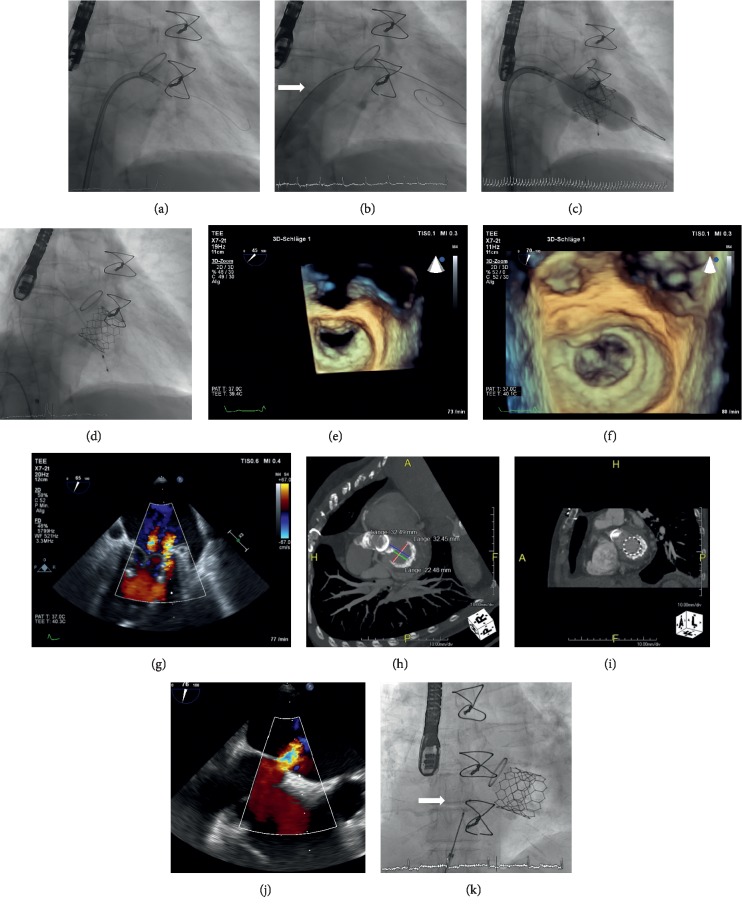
TMVI-MAC: (a) fluoroscopy shows a 0.035-inch J-guide inserted through the mitral valve in the left ventricle via a steerable guiding catheter (Agilis, 8,5F, Abbott, Illinois, USA). (b) Fluoroscopy shows balloon dilatation (Osypka VACS II 12 × 60 mm Balloon, Osypka AG, Germany, white arrow) of the interatrial septum over an Amplatz Super Stiff guidewire (Boston Scientific) to create an artificial atrial septum defect prior to insertion of the prosthesis into the left atrium. (c) *Fluoroscopy* shows the implantation of a 29 mm Edwards SAPIEN 3 (ES 3) prosthesis into the heavily calcified native mitral valve. (d) *Fluoroscopy* shows the final result after implantation of the ES 3 prosthesis into the calcified native mitral valve. (e) 3D-*TEE* shows the native mitral valve with a heavily calcified native mitral ring prior to TMVI (view from left atrium). (f) 3D-TEE shows the result after TMVI of the 29 mm ES 3 prosthesis into the advanced mitral annular calcification (MAC). (g) 2D-*TEE* with color duplex shows only minimal paravalvular and valvular regurgitation after implantation of the ES 3 Prosthesis into the MAC. (h) Cardiac CT-scan shows the exact dimensions of the calcified mitral valve prior to implantation. (i) Cardiac CT-scan shows the final result after TMVI into MAC. (j) 2D-TEE with color duplex shows a large iatrogenic atrial septal defect (ASD) with clinically relevant right-to-left cardiac shunt. (k) *Fluoroscopy* shows successful transcatheter implantation of an Amplatzer Septal Occluder (Abbott, Illinois, USA) into the ASD (white arrow).
